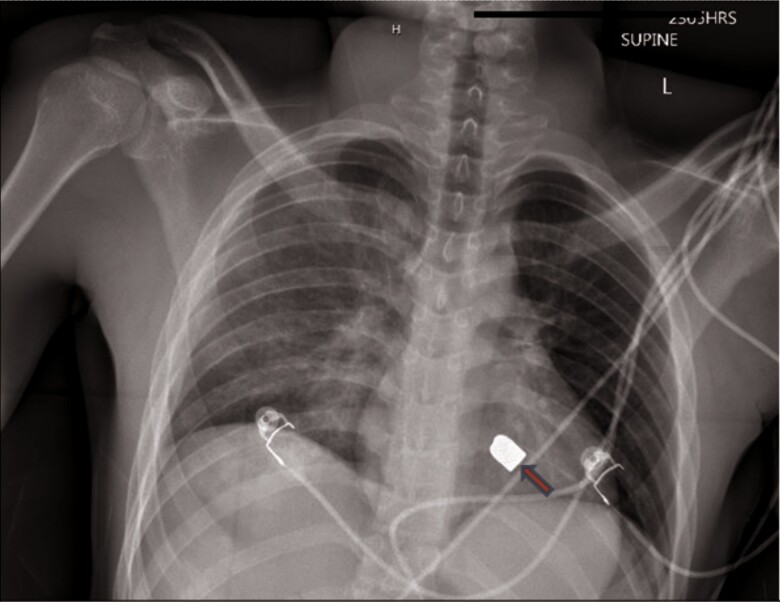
Chest X-ray at the time of arrival to the ED demonstrated a metallic foreign body consistent with a bullet overlying the cardiac silhouette.
